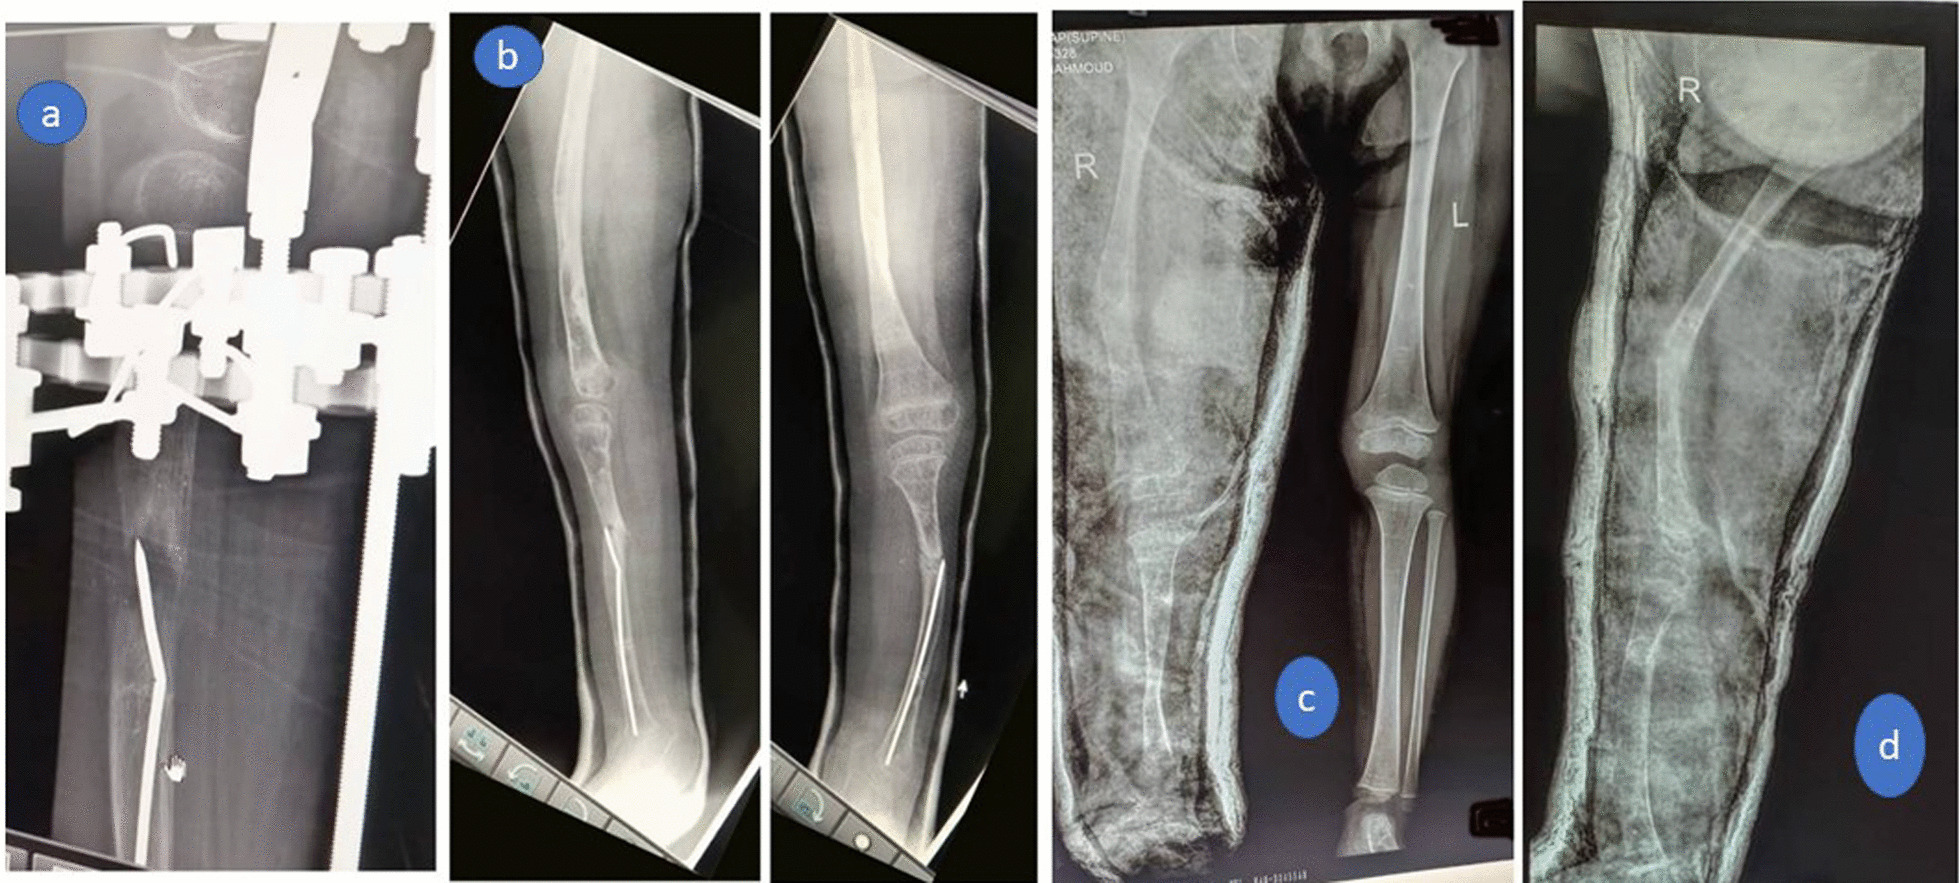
a Valgus deformity occurred at the tibial regenerate, which was managed by b corrective osteotomy and intramedullary rodding, c anteroposterior, and d) lateral x-ray images of Insufficiency fracture of the femur at the site of a previous pin
